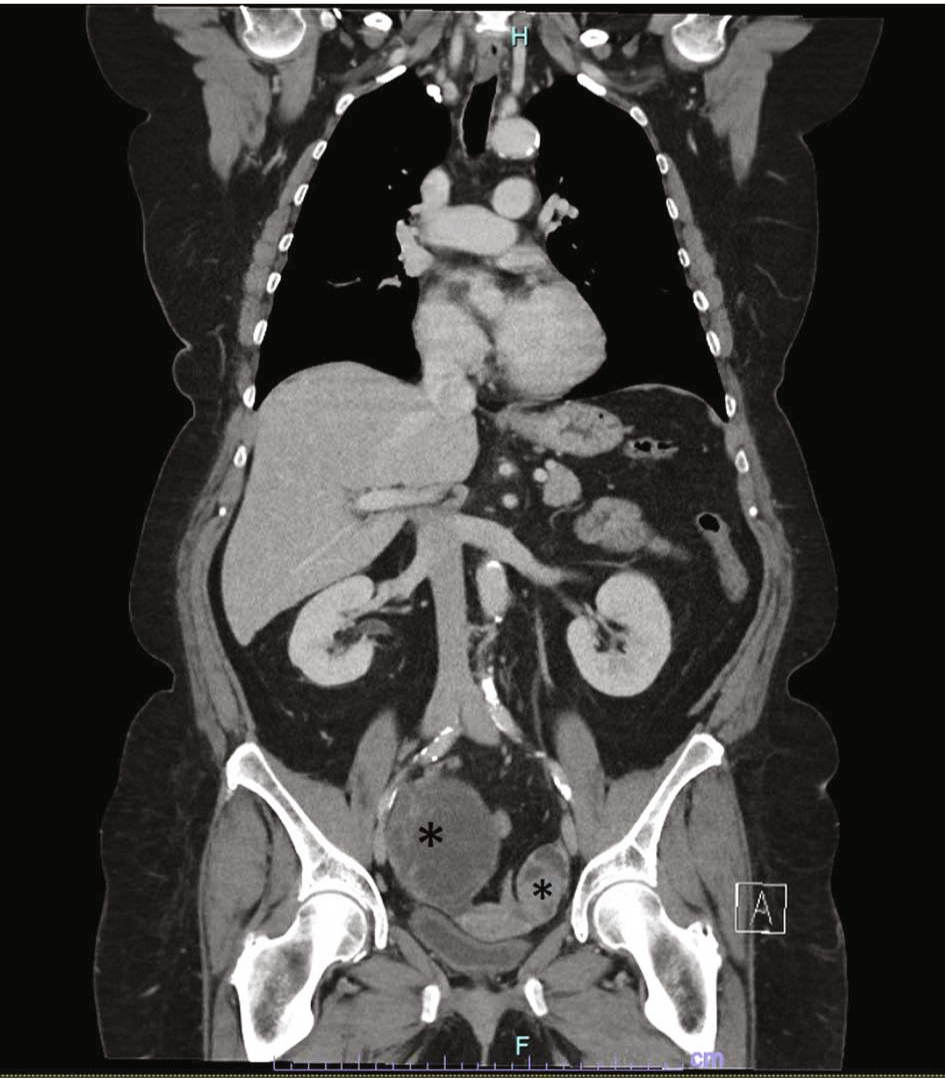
CT A/P. The asterisks mark the enlarging right ovarian lesion and a new left ovarian lesion.
